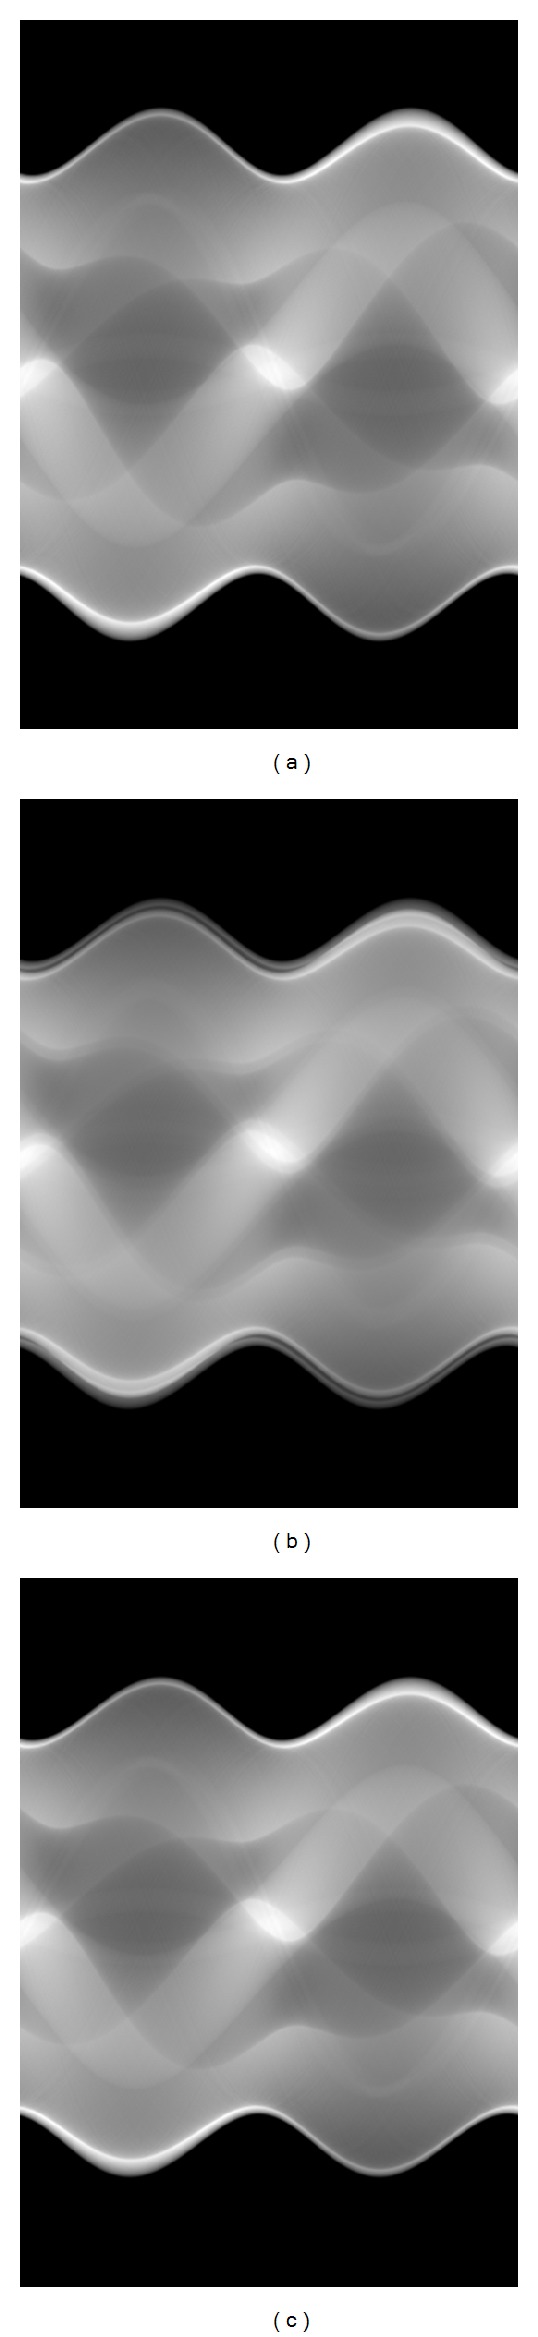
Sinogram of projections: from left to right it is under single X-ray source, overlapped and separated under double X-ray sources.
